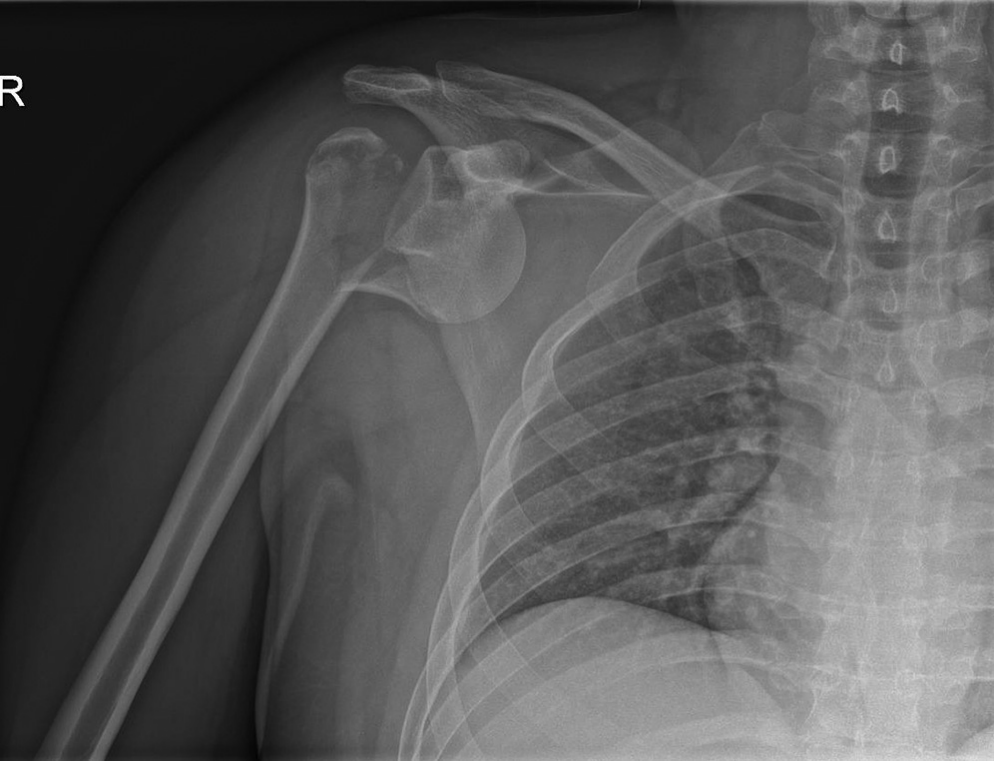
Radiograph of the preoperative shoulder showing a comminuted fracture-dislocation of the proximal humerus on the *right* side.
